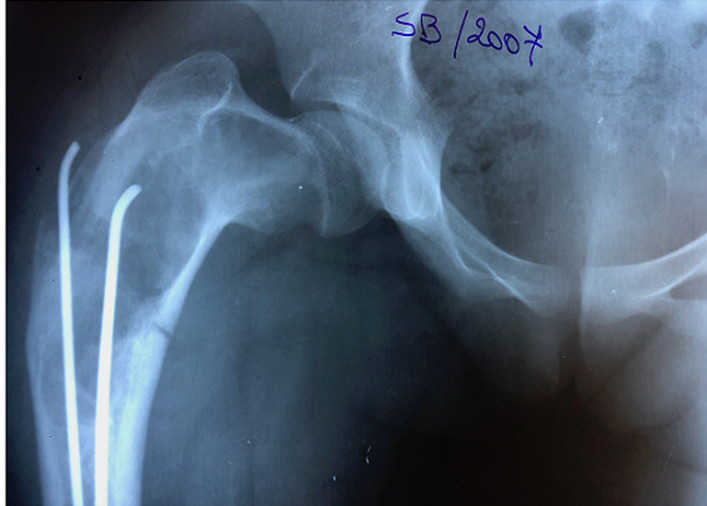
image radiographique montrant une fissure de contrainte au niveau de la partie inférieure du col fémoral
